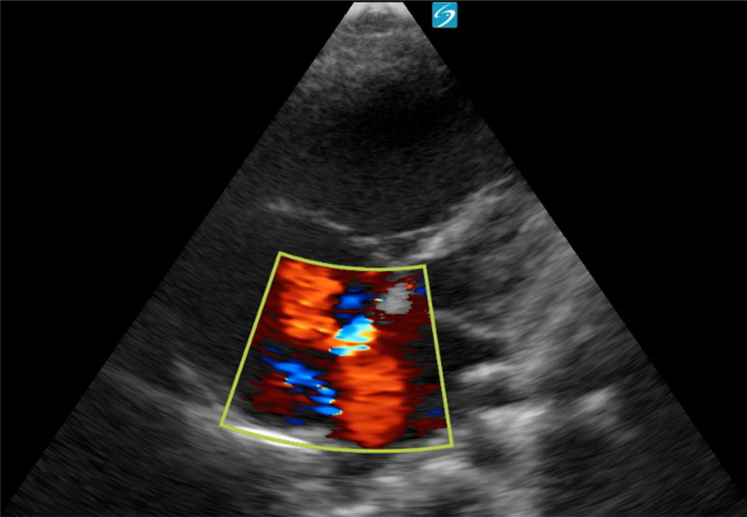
Apical four-chamber view with color Doppler demonstrating left-to-right shunting through an atrial septal defect. Image taken by the author.
